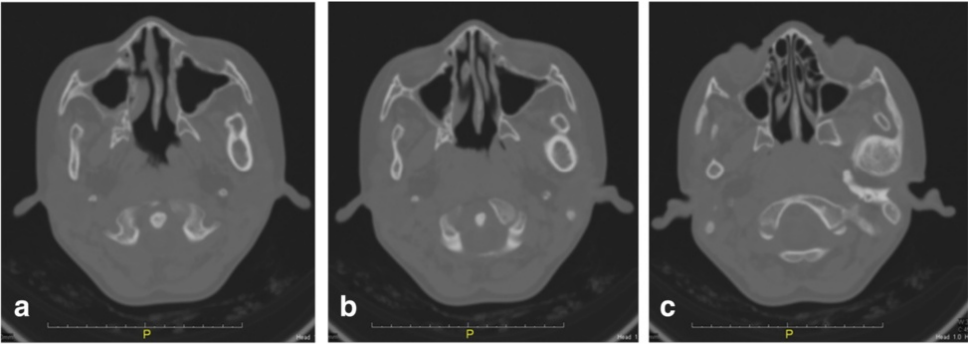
a, b and c: Axial views of CT scan, showing the bony expansion medio-lateraly from the left ascending ramus to the condylar head
